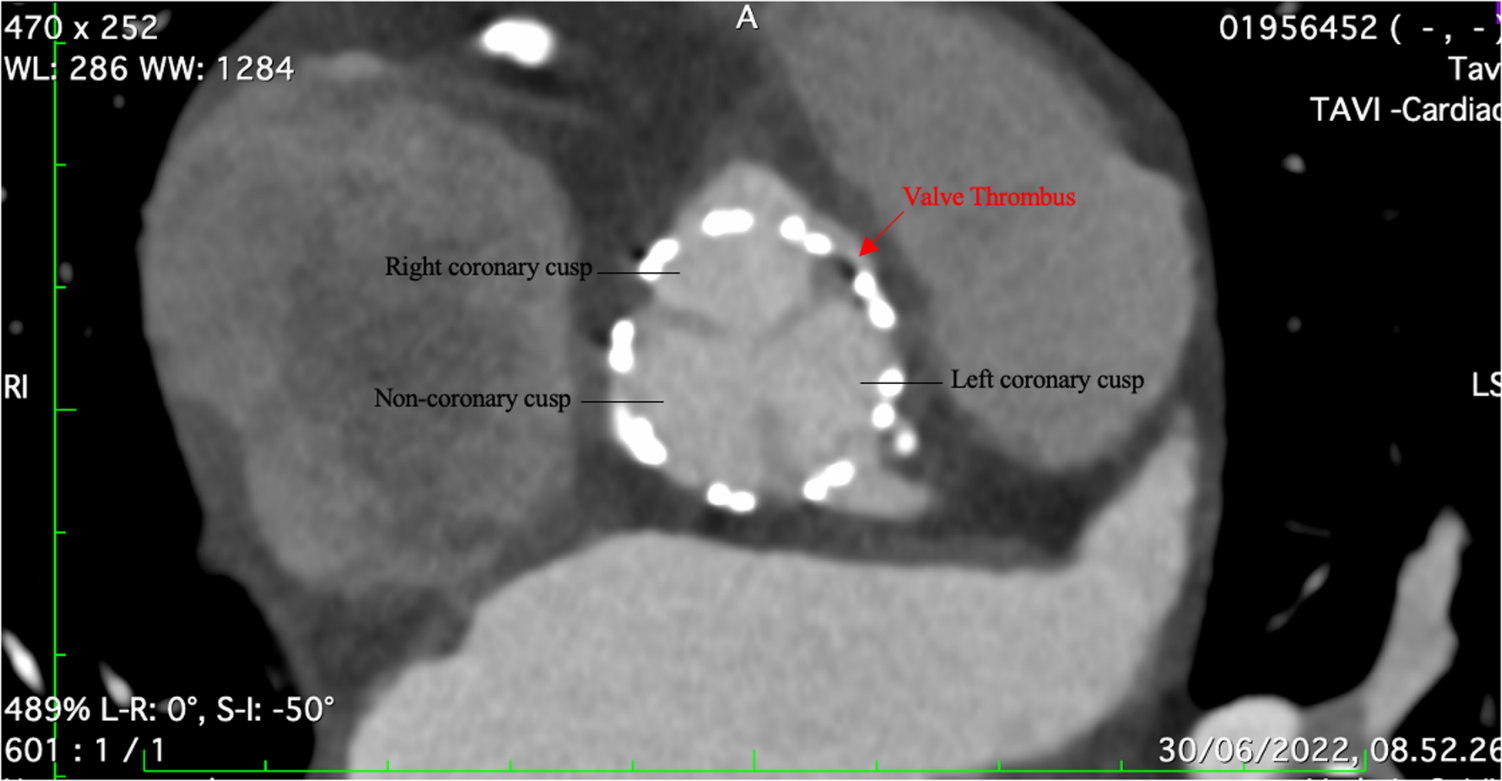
CT angiography showing a small thrombus between the right coronary and left coronary cusp.
